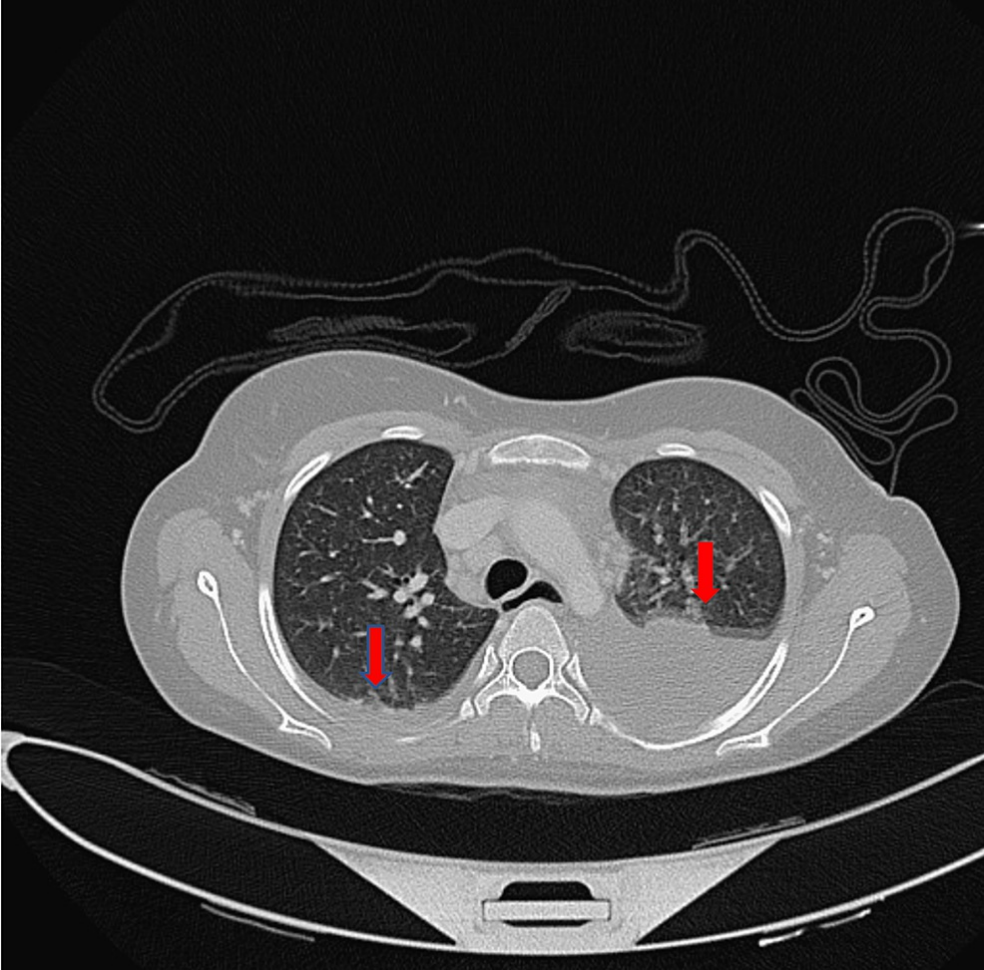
Chest CT It showed bilateral pleural effusion (red arrows) associated with bilateral lower lobe lung collapse. Pericardial effusion was also observed. CT, computed tomography.
